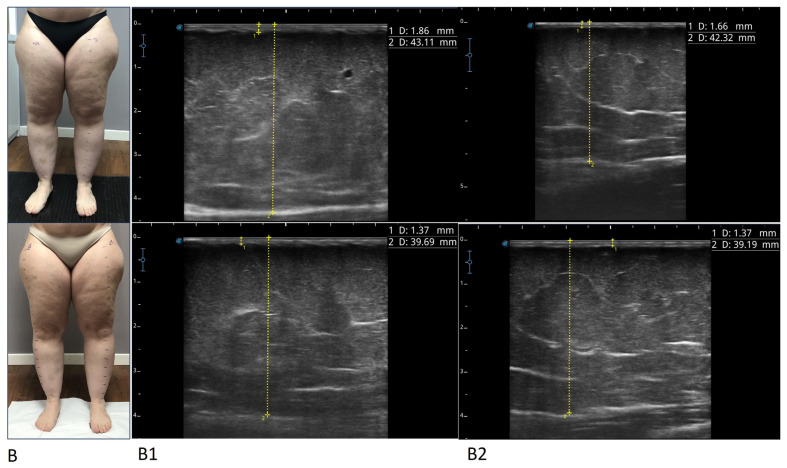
Photographs and ultrasounds of two patients (A,B), before treatment (images above) and after treatment (images below). The ultrasound measurements are represented by the dashed yellow lines: the thickness of the skin was measured from the skin surface to the lower edge of the epidermis and dermis complex, and the thickness of the suprafascial superficial adipose tissue was measured from the skin surface to the subcutaneous transition structure (fascia). All imaging was performed using a high-frequency linear probe (8–14 MHz), keeping the probe perpendicular to the skin and always without applying pressure. For patient A, ultrasound images relating to the lower medial third of the leg (A1) and the upper lateral third of the leg (A2) before and after the treatment are shown (box above and box below, respectively). For patient B, ultrasound images relating to the lower medial third of the thigh (B1) and the upper medial third of the thigh (B2) before and after the treatment are shown (box above and box below, respectively).
